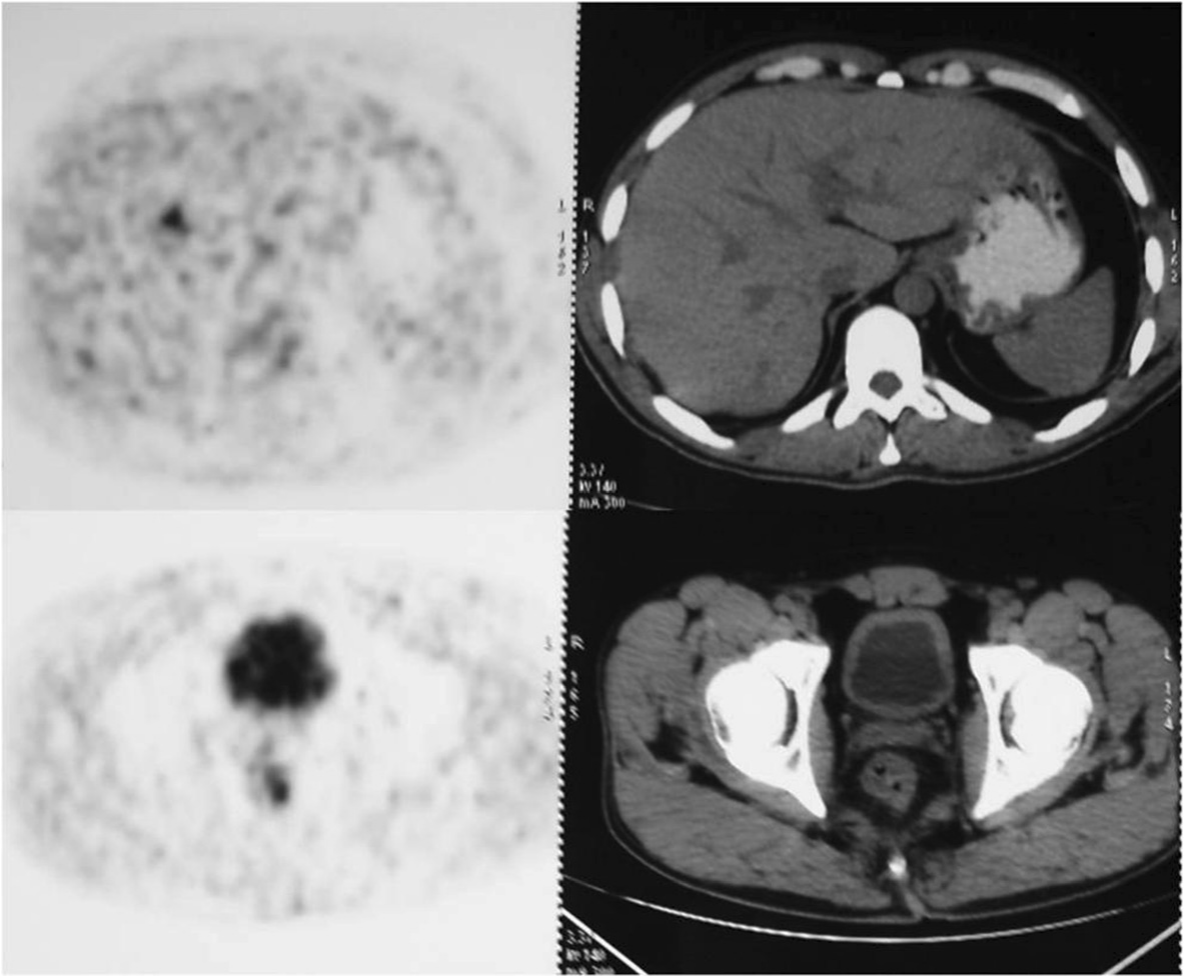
Appearance of primary tumor and hepatic metastases in PET/CT image.
